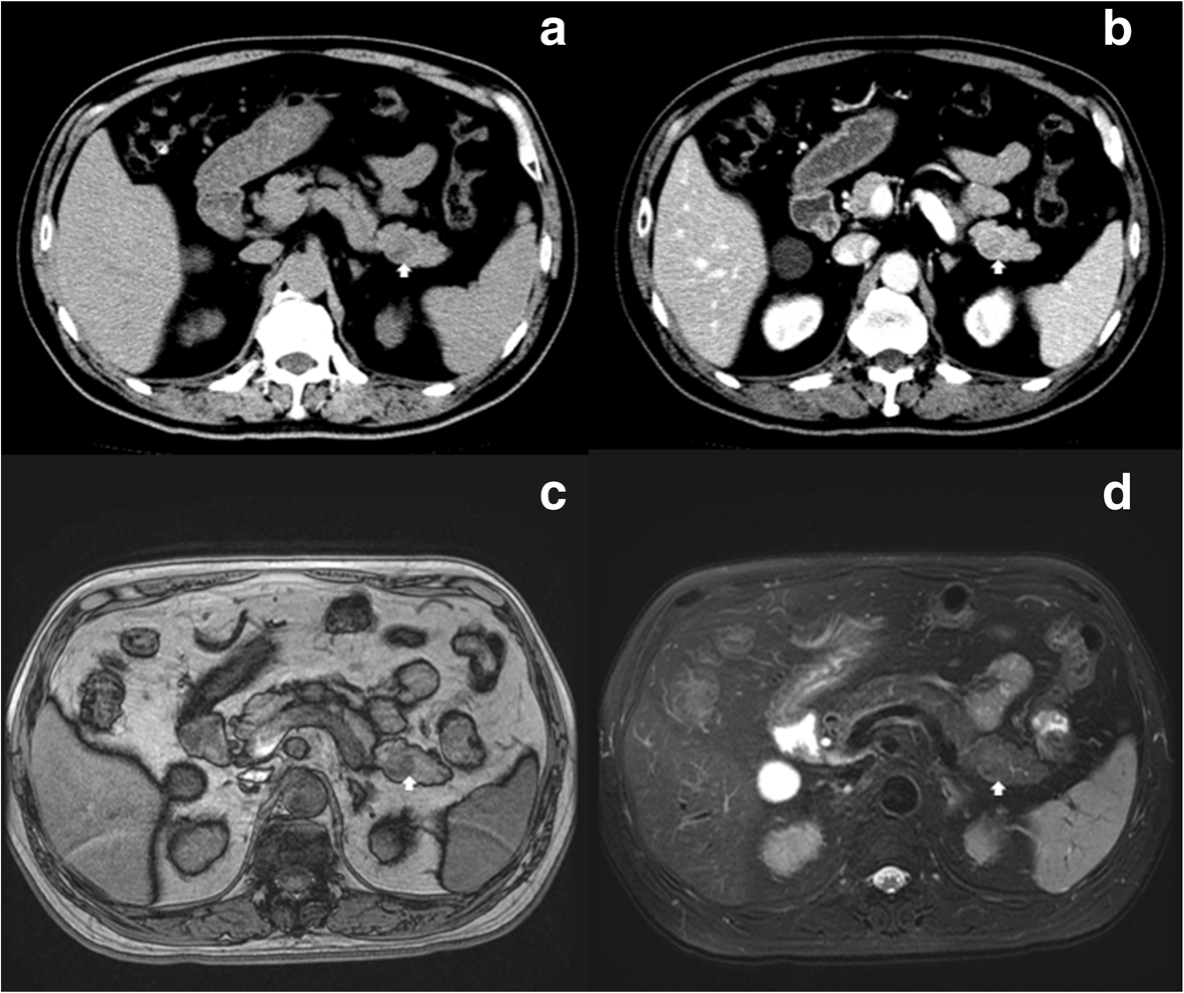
a A heterogeneous hypodense nodule (*white arrow*) is depicted at pancreatic tail in pre-contrast CT; b After contrast administration, a well-enhanced tumor with pseudocapsule (*white arrow*) is identified; c The nodule shows signal drop (fat) on T1 opposed phase MRI image, probably due to fatty metamorphosis (*white arrow*); d However, the lesion is isosignal (no water) on T2 weighted MRI image (*white arrow*)
